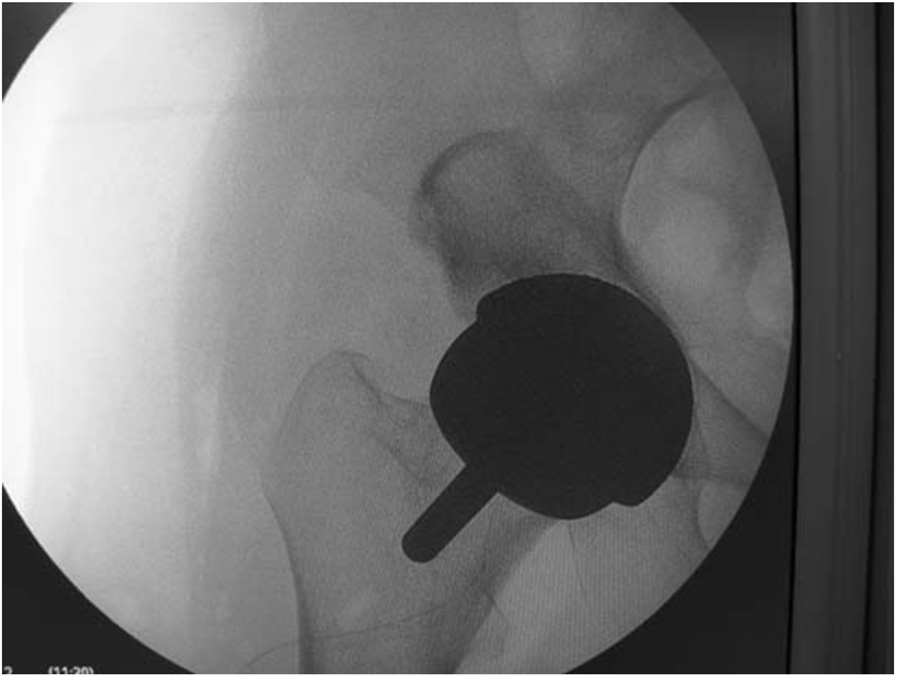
The X-ray plain film of prosthesis location. The X-ray plain film shows that the location of prosthesis is good during the operation. Postoperative radiograph showing typical hip resurfacing arthroplasty in the anteroposterior view
